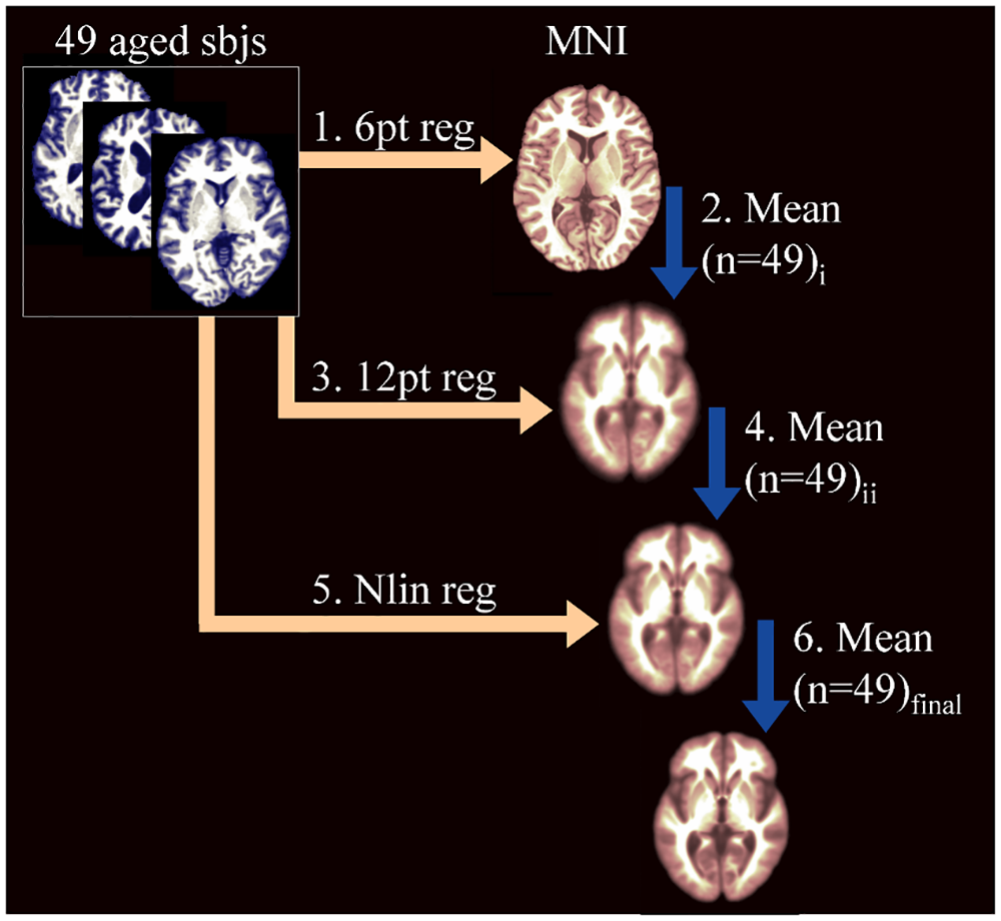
Procedure to create a brain MRI standard space representative of aged (>60 years) subjects. This is in the orientation of the commonly used Montreal Neurological Institute (MNI) template but reflects features of brain ageing, e.g. larger ventricles; sbjs = subjects; pt = point; reg = registration; Nlin = nonlinear.
